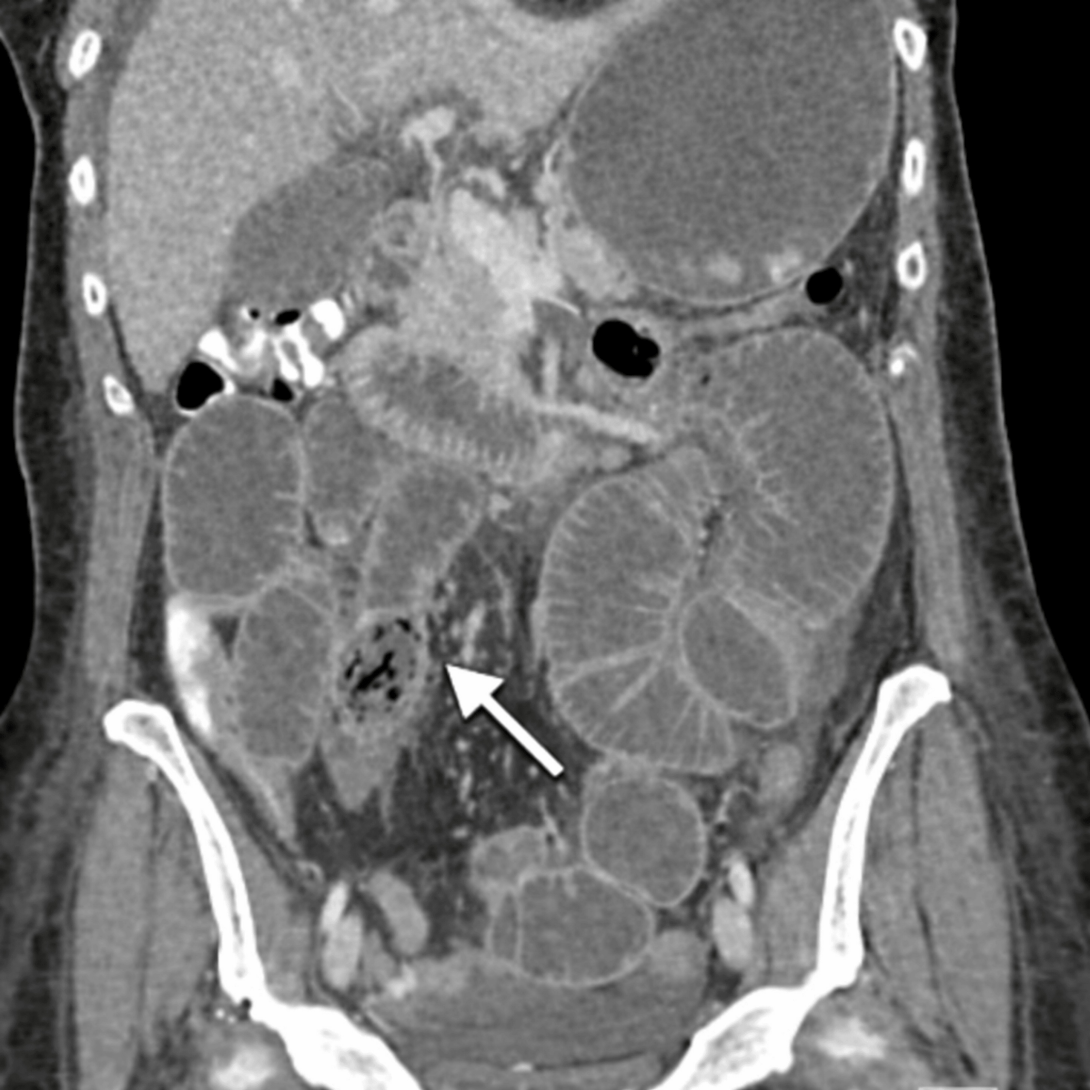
Coronal contrast-enhanced CT image demonstrating small bowel obstruction with a discrete transition point caused by an obstructing intraluminal mass with a heterogeneous internal appearance (arrow).
